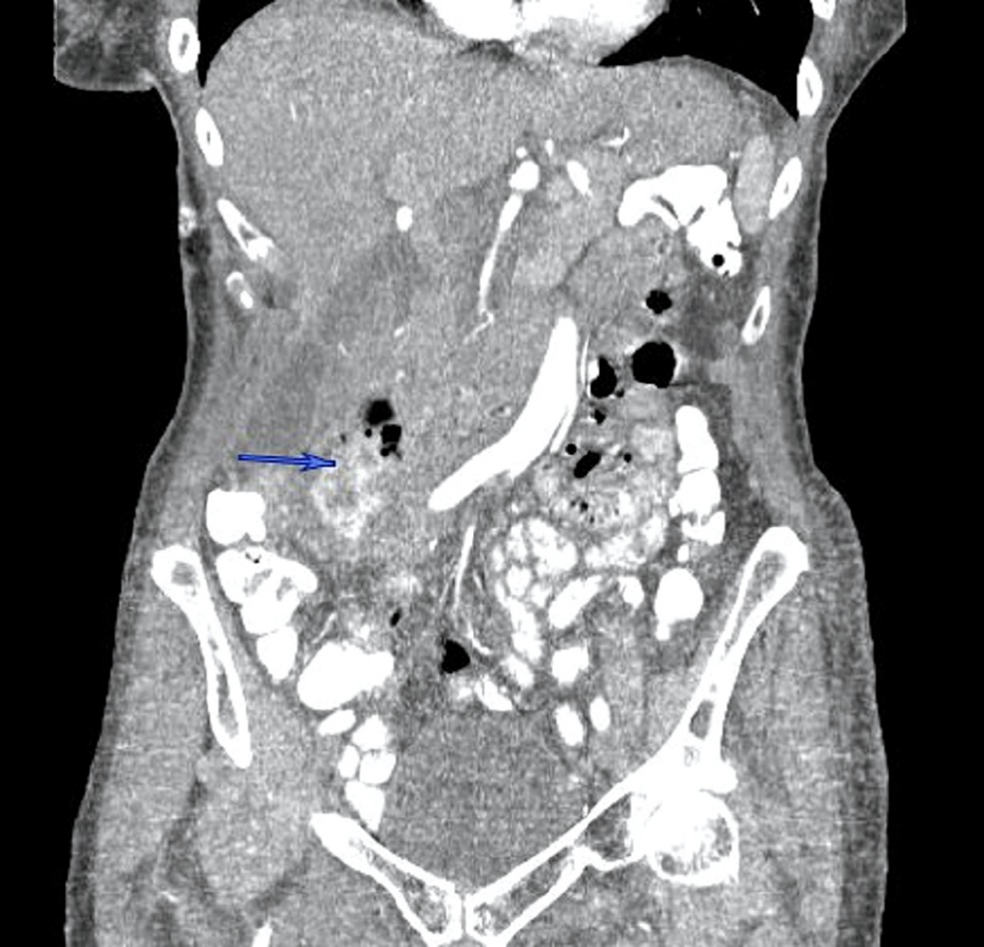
CT abdomen (coronal section) demonstrates a complex collection with a contained contrast leak related to the perforated duodenal diverticulum
